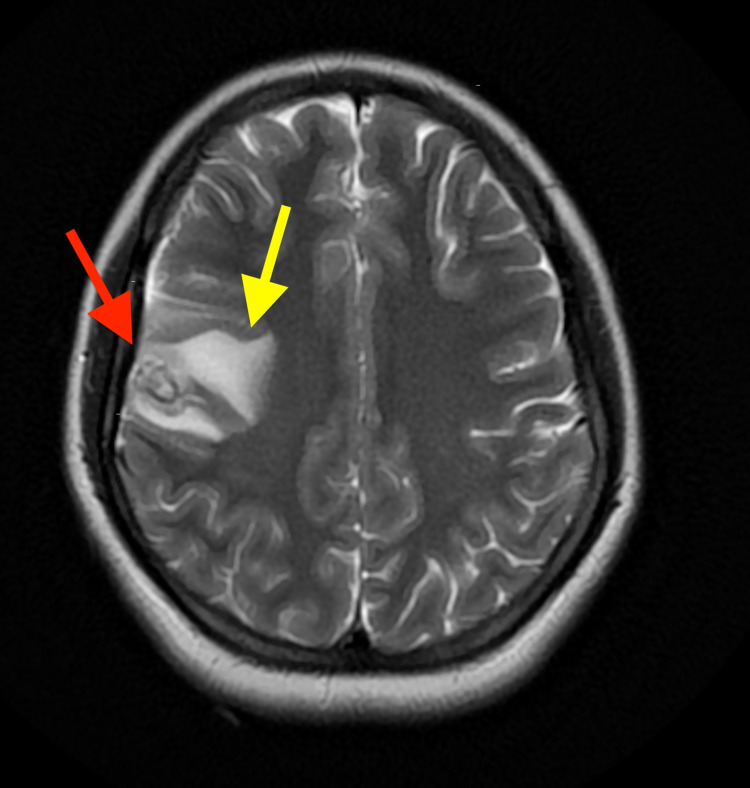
Axial view MRI brain showing solitary cystic mass (red arrow), with surrounding vasogenic edema (yellow arrow).
